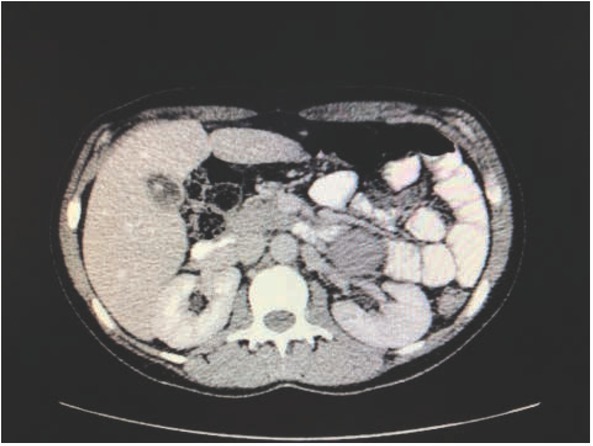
CT scan with suspected pancreatic tumor
